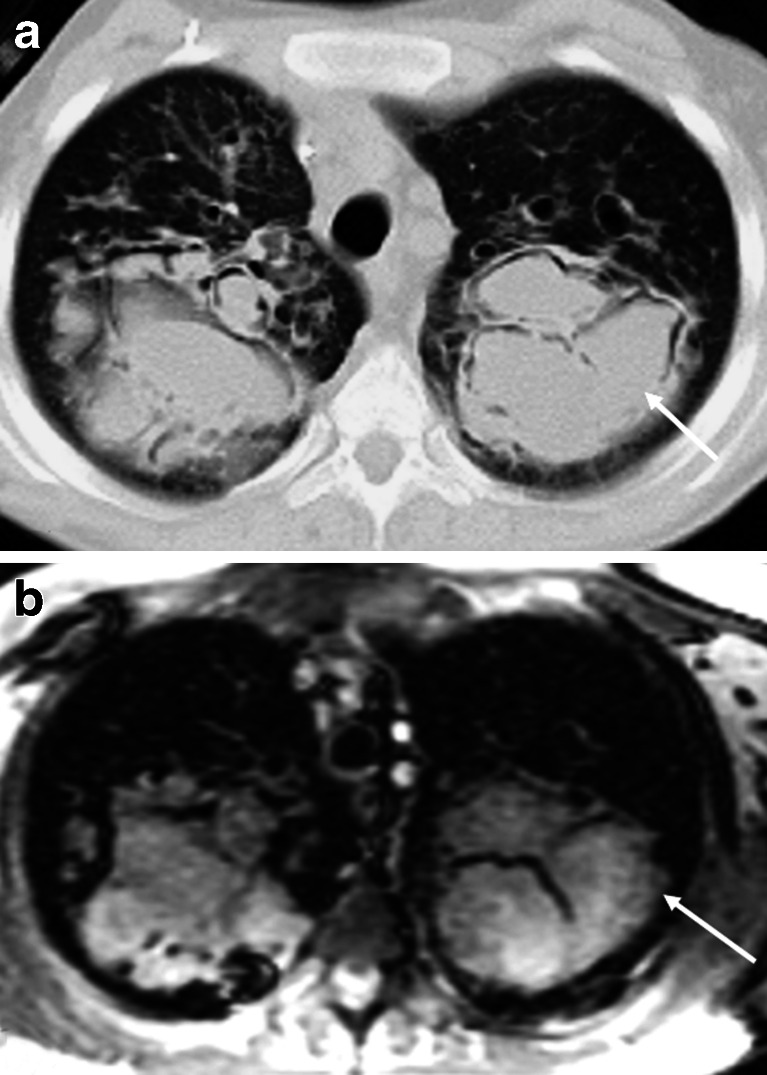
Aspergilloma in cystic fibrosis. a CT versus (b) steady-state free precession MRI in a 16-year-old boy with end-stage cystic fibrosis. Note widely dilated bronchi filled with thick mucus and aspergillomas (*arrows*)
